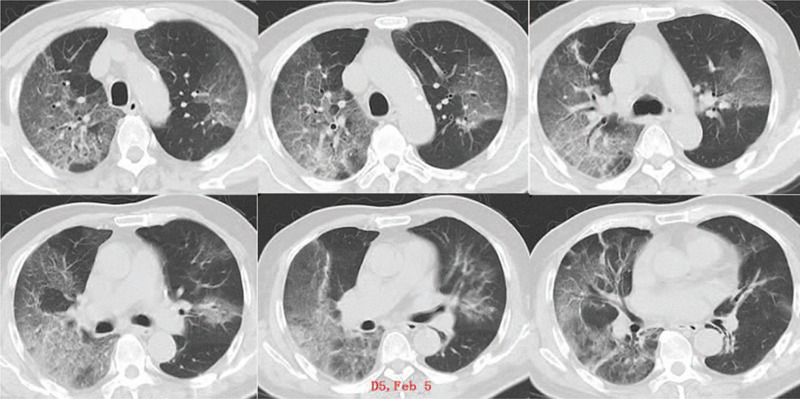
Chest CT images of the patient at admission on February 5, 2020 (D5).
